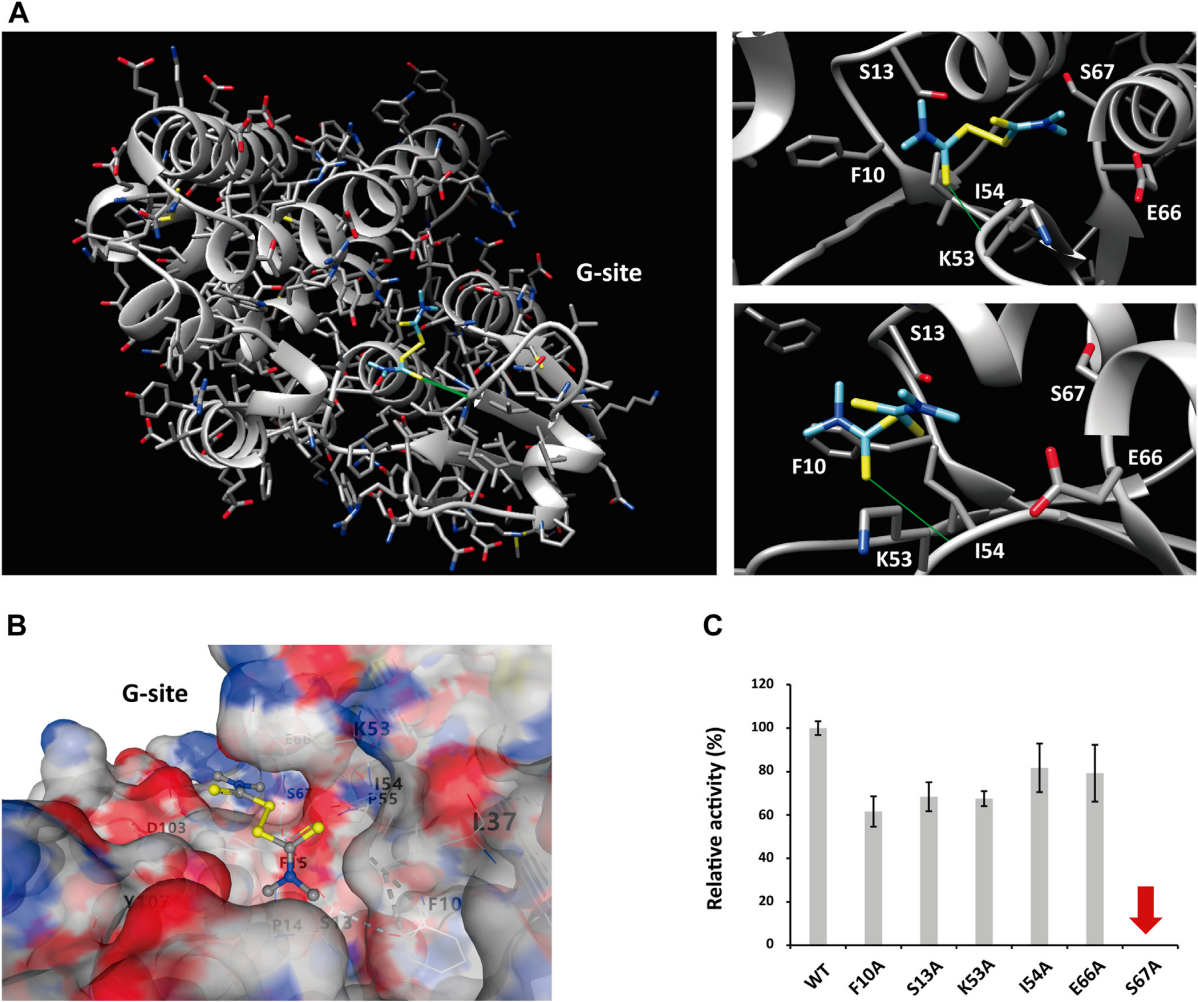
TCpGST structural analysis and residues involved in the enzymatic reaction.*A*, interactions of thiram (1) with the residues in the active site of TCpGST. The three-dimensional structure of TCpGST was predicted using Phyre2 according to the reported X-ray three-dimensional structure of the tau class GST from *Glycine max* (https://www.ebi.ac.uk/pdbe/entry/pdb/2vo4) (28). The docking study was performed using SwissDock (56). *B*, conformation of 1 in the G-site of TCpGST. The location of 1 in the G-site of TCpGST was calculated using the CB-Dock software (55). *C*, activity study of WT and TCpGST active site mutants toward 1. The mutants were constructed by replacing residues F10, S13, K53, I54, E66, and S67 with alanine. Mutant S67A did not show degrading activity toward 1. TCpGST, tau class glutathione *S*-transferase from *Carica papaya*.
